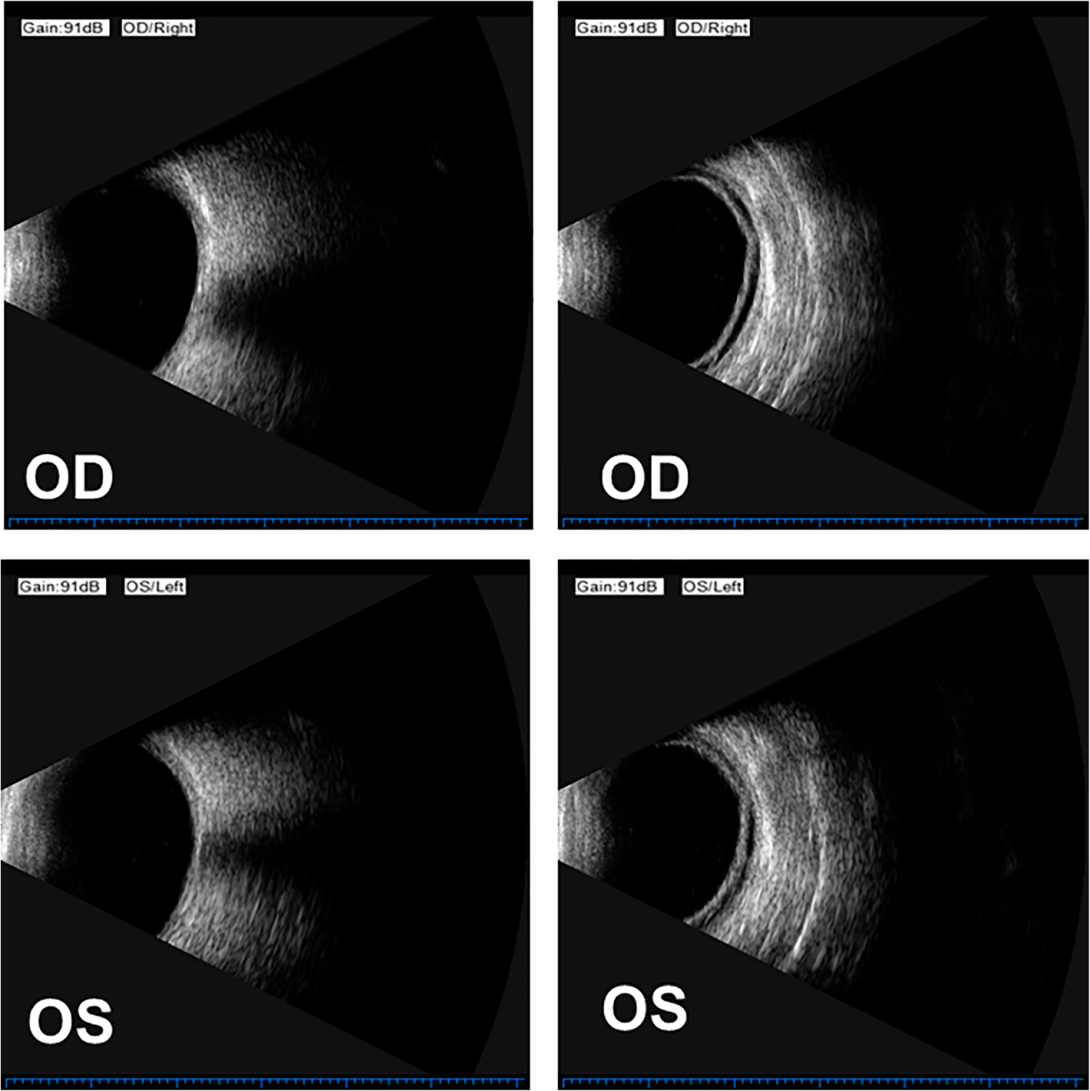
Ultrasonography showed that there were no special findings in vitreous cavity and posterior wall of the ball in both eyes, but the highly reflective band was separated from the wall in the periphery
